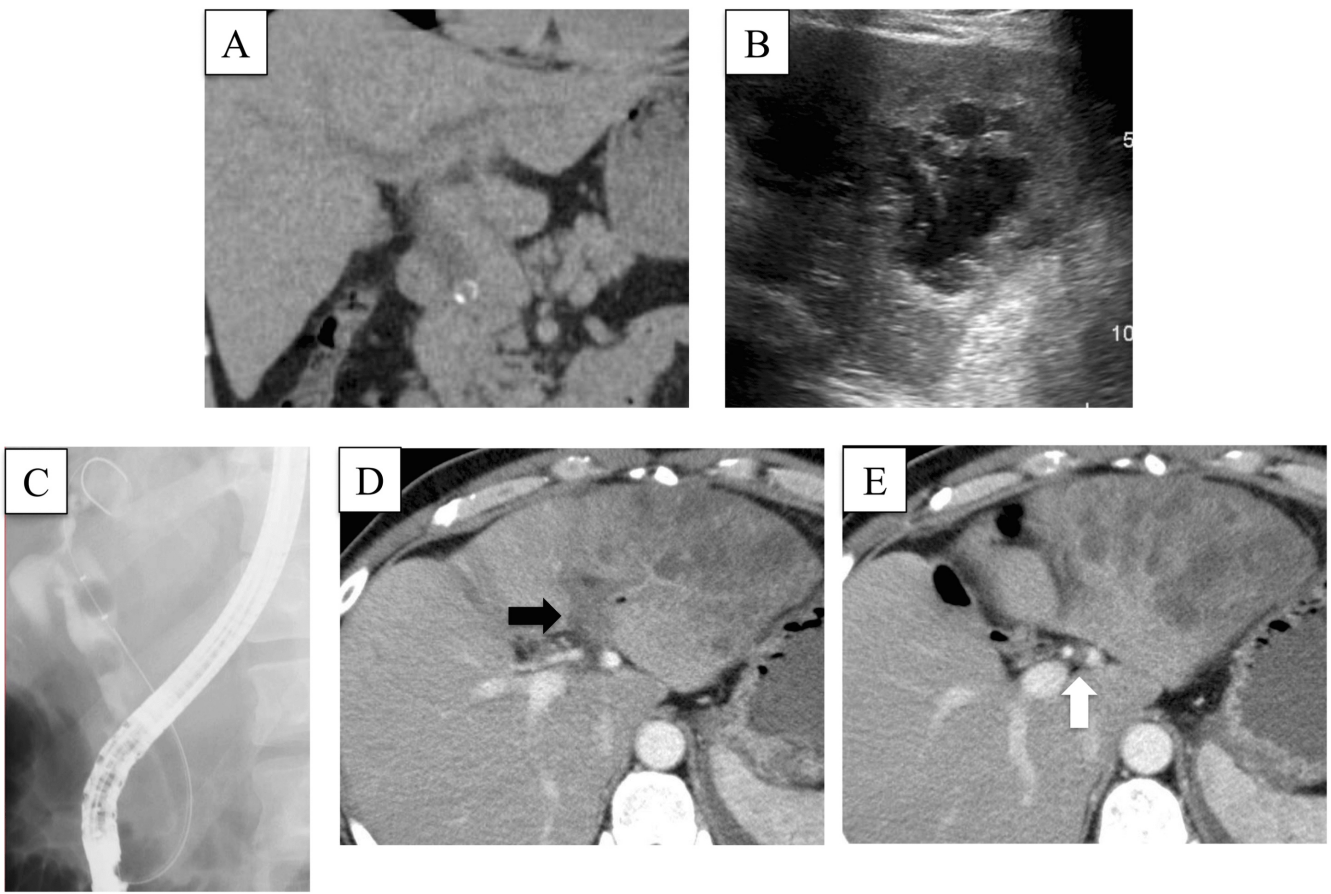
Inspection results (A) Computed tomography image revealing dilated bile ducts proximal to obstructing common bile duct stones; (B) Ultrasound image showing a multilocular, low-echoic, 8-cm lesion diagnosed as a left hepatic lobe abscess; (C) Endoscopic retrograde cholangiopancreatography image showing stones in the common bile duct with dilatation; (D and E) Contrast-enhanced CT scan images revealing that the left branch of the portal vein (black arrow) and the left hepatic artery (white arrow) are obstructed proximal to the infarction.
